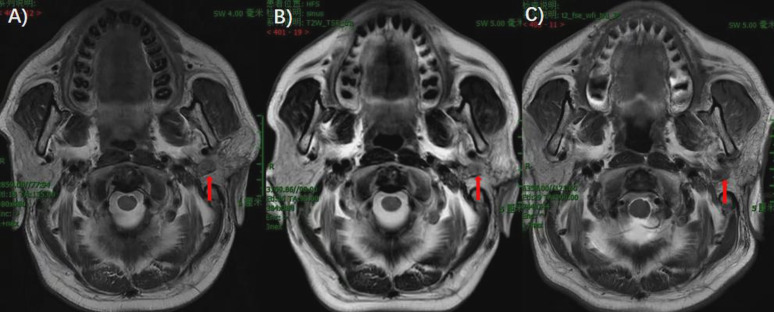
T2-weighted MRI follow-up findings. (A) During radiotherapy (after 13 fractions); (B) One month post-radiotherapy; (C) Three months post-radiotherapy.
